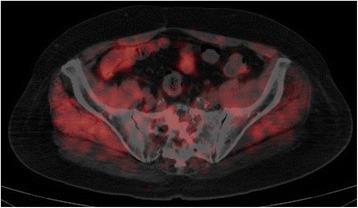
PET-CT 2 months after the second course of heavy ion radiotherapy. PET-CT shows disappearance of the abnormal accumulation in the sacral lesion 2 months after the second course of heavy ion radiotherapy
